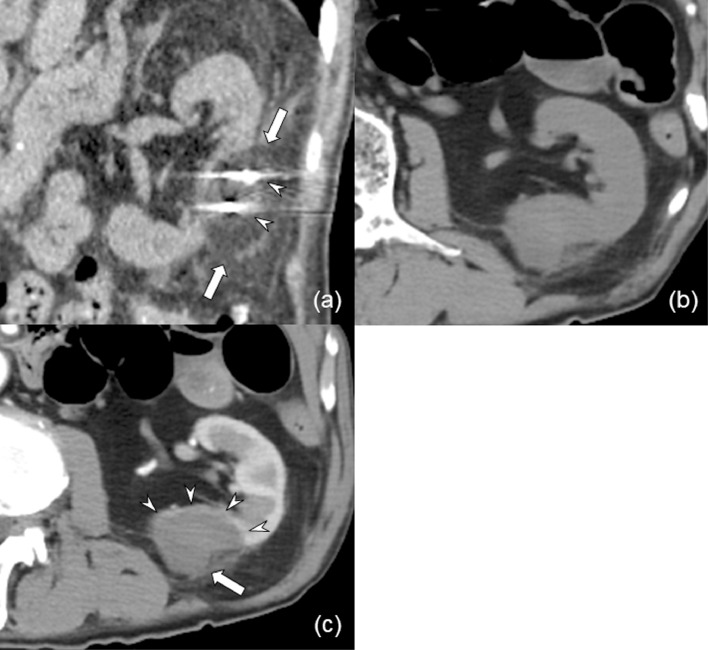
Sagittal reconstructed computed tomography (CT) image during initial cryoablation (CA). The renal mass on the left kidney is covered with low attenuation ice ball (arrows) with a sufficient margin. Arrowheads show 2 cryoprobes. (B, C) CT images obtained 2 weeks after initial CA. Contrast-enhanced CT image shows no enhancement of the mass (arrow) or ablation margin (arrowheads).
